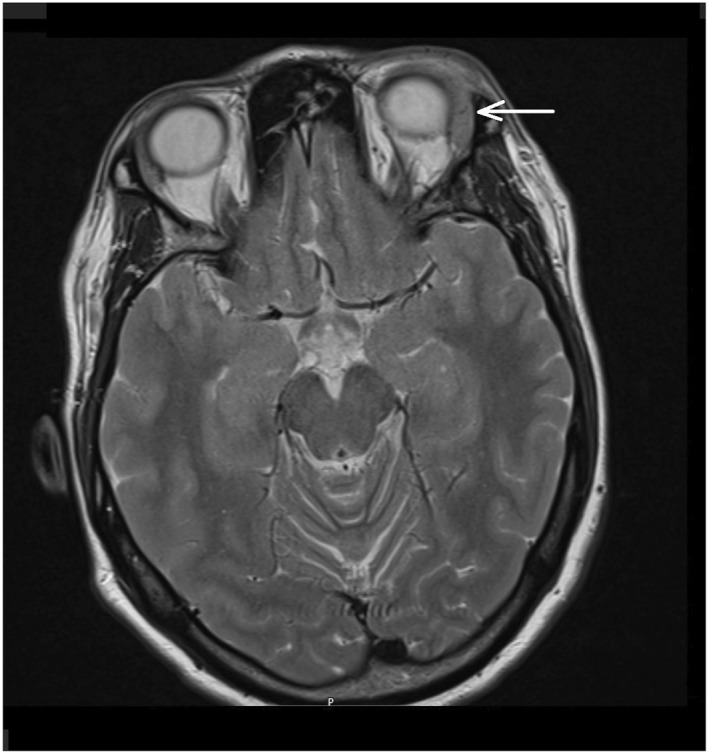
MRI T2‐weighted, axial plane. Enlargement of left lacrimal gland.
